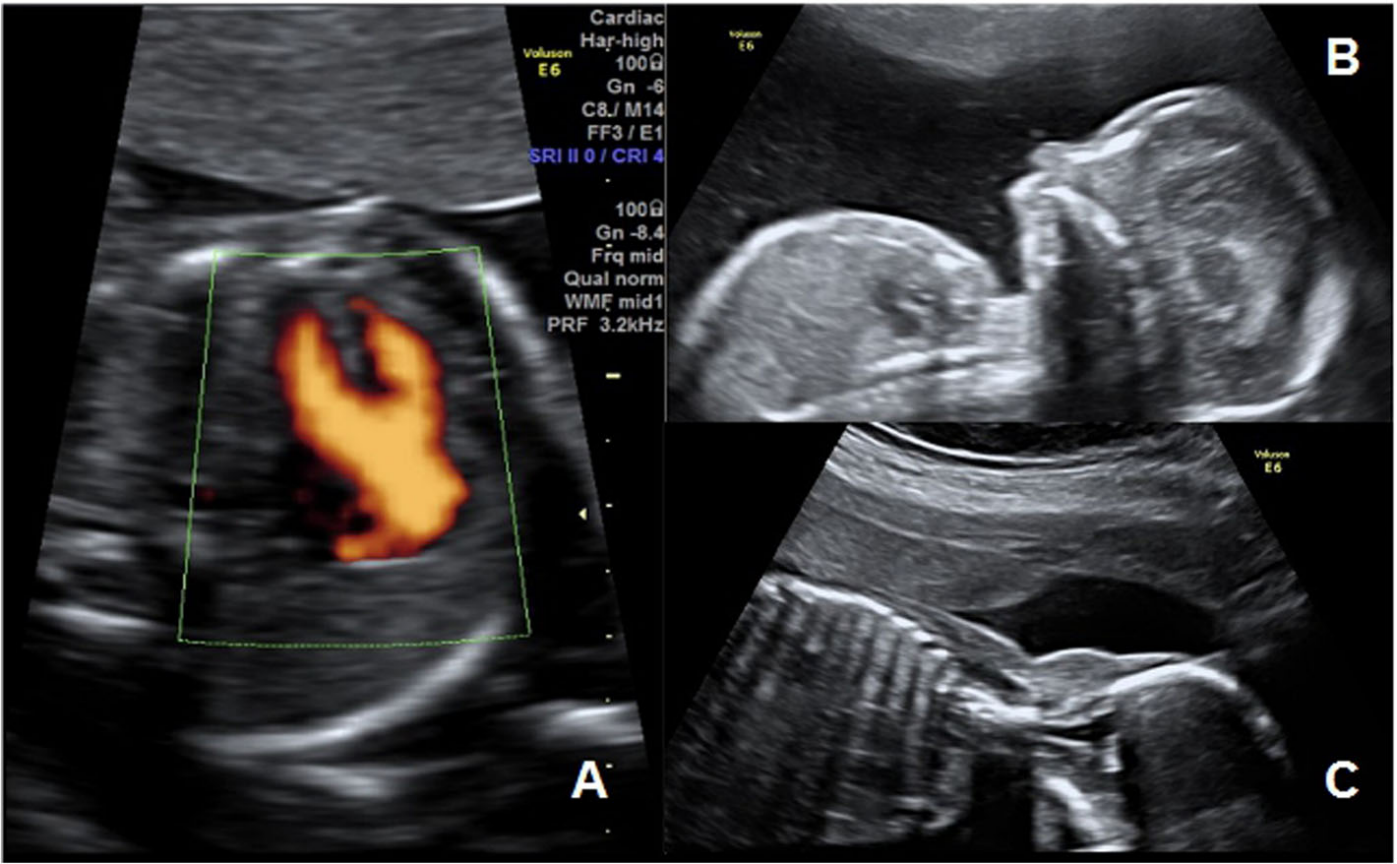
Case 1 mid-trimester ultrasound scan. a single great artery that equal overrode a ventricular septal defect; b microretrognathia; c nuchal edema
